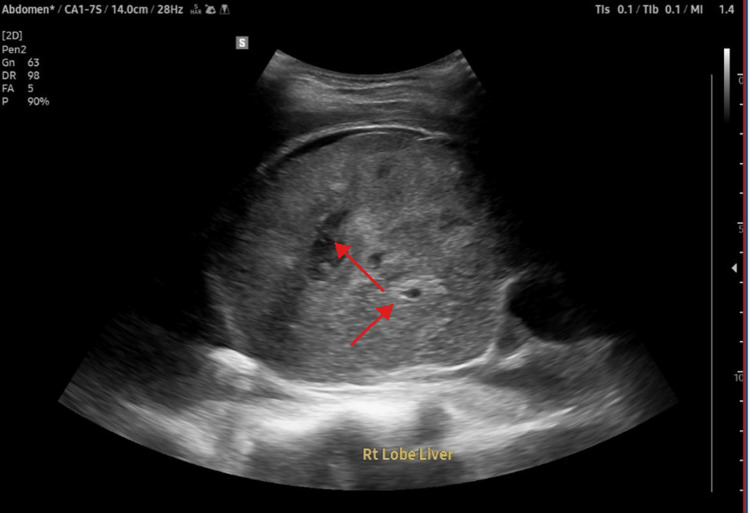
Initial abdominal ultrasound (prior to aspiration) demonstrating the large, well-defined 12.5 x 9 cm cystic lesion in the right lobe of the liver
